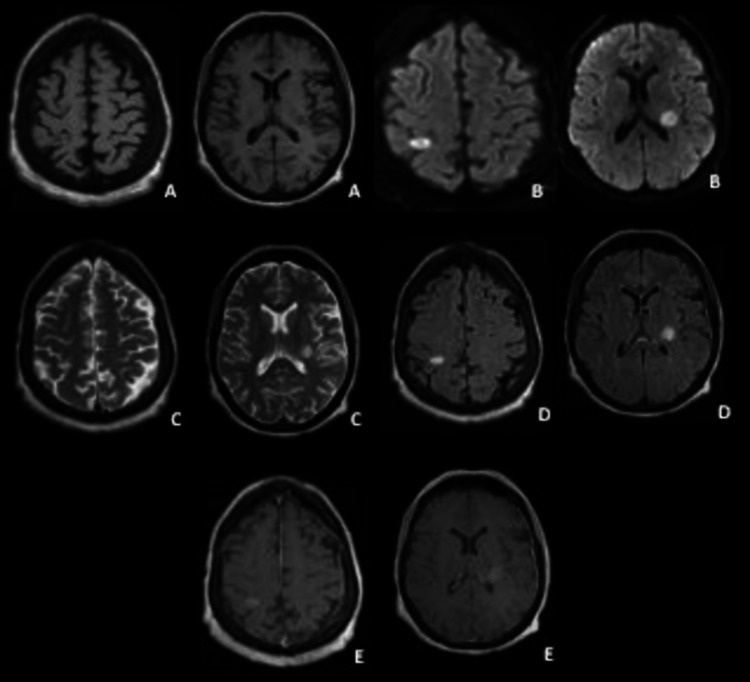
Brain magnetic resonance imaging (1.5 Tesla) A) T1 axial view; B) Diffusion axial view; C) T2 axial view; D) T2 fluid-attenuated inversion recovery (FLAIR) axial view; E) T1 axial view with gadolinium revealed right parietal nodular signal abnormality in the posterior limb of the internal capsule with enhancement after gadolinium
